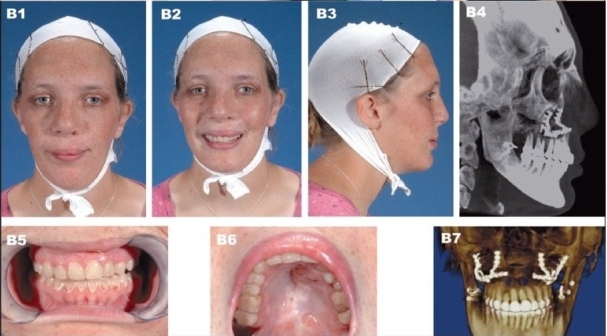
(B) Patient at 19 years of age demonstrating long-term outcome after two-piece Lefort I osteotomy with maxillary advancement, bilateral mandibular ascending ramus sagittal split osteotomy, and onlay demineralized bone grafting to bilateral cheeks. (B1) Frontal view (B2) Frontal smiling (B3) Right lateral view (B4) Postperative lateral cephalometric roentgenogram (B5, B6) Occlusion after completion of treatment (B7) 3D CT image showing the final occlusion and internal semi-rigid fixation
